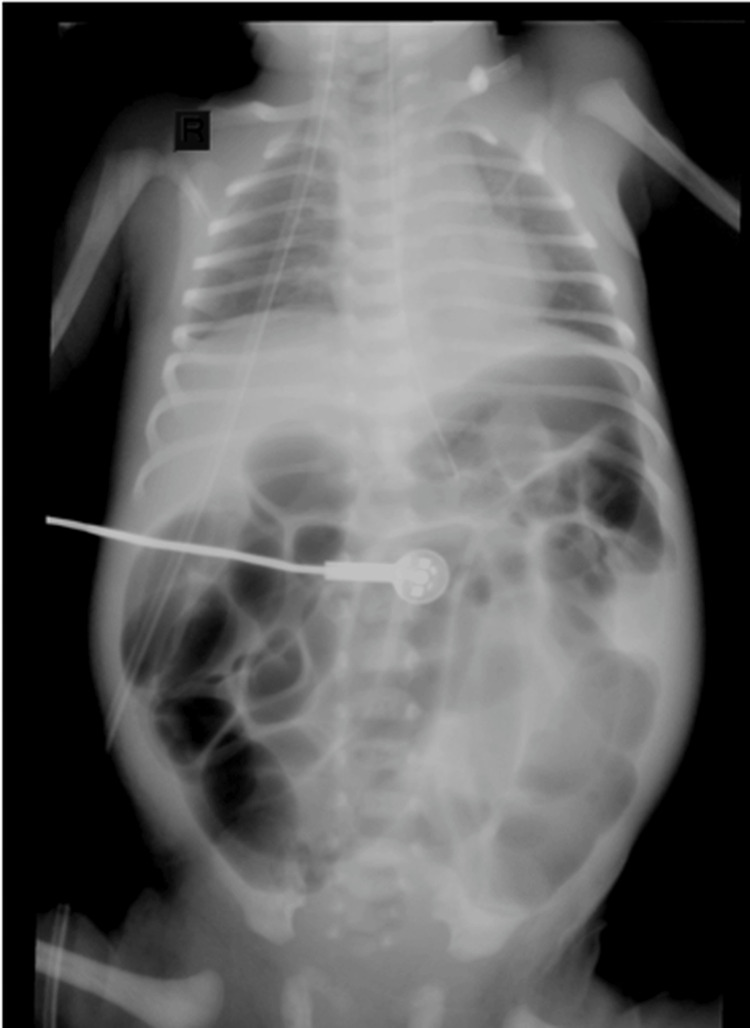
Abdominal X-ray demonstrating diffuse distension of intestinal loops, consistent with distal bowel obstruction in the context of Hirschsprung disease
